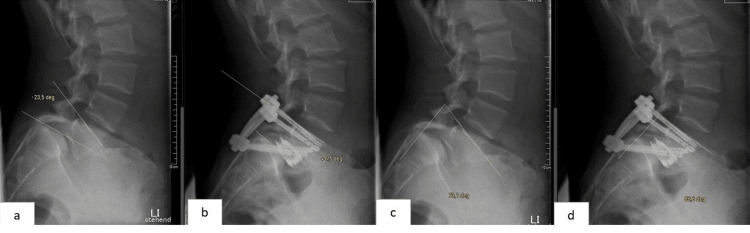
Preoperative and postoperative standing X-ray views showing a 30° reduction of slip angle (a and b) and preoperative and postoperative standing X-ray views showing a 13.5° reduction of lumbosacral angle (c and d).
